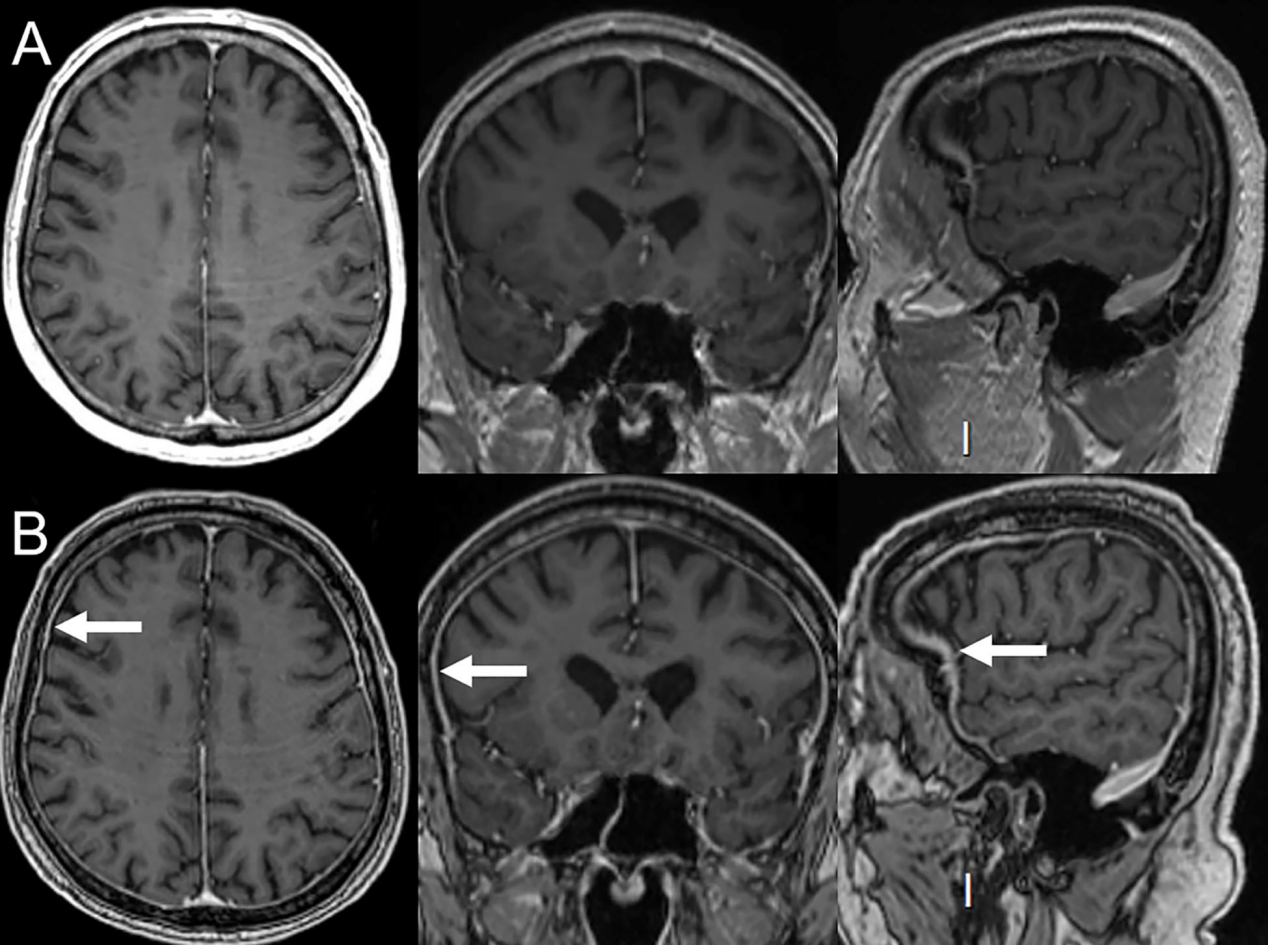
Immune-related aseptic meningitis in a 78-year-old female with NSCLC treated with ipilimumab (anti-CTLA-4) and nivolumab (anti-PD-1). (A) Normal brain MRI (axial, coronal and sagittal plans) performed 6 months prior to immunotherapy. After 3 cycles of ipilimumab and nivolumab, the patient developed headaches. (B) MRI performed 2 months after the beginning of immunotherapy showed smooth diffuse dura mater thickening (arrows) compatible with aseptical meningitis. Patient’s symptoms and signs of inflammation on MRI disappeared upon immunotherapy discontinuation.
